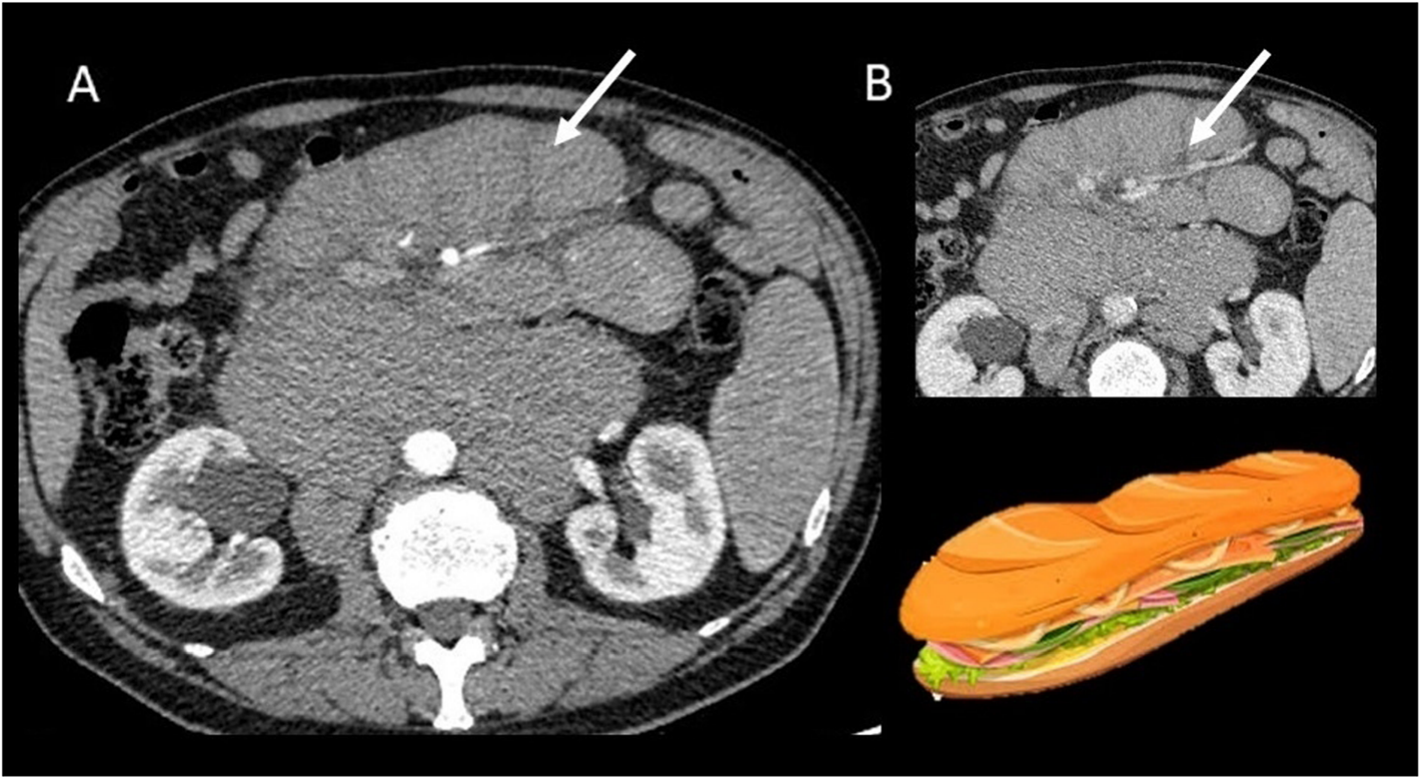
Patient affected by non-Hodgkin lymphoma with both retroperitoneal and mesenteric locations. CT images show a large lesion with well-defined margins and homogeneous enhancement both on arterial (a) and portal (b) phases. Confluent lymphadenopathy on both sides of the mesenteric vessels gives rise to an appearance described as the sandwich sign that is specific for mesenteric lymphoma
